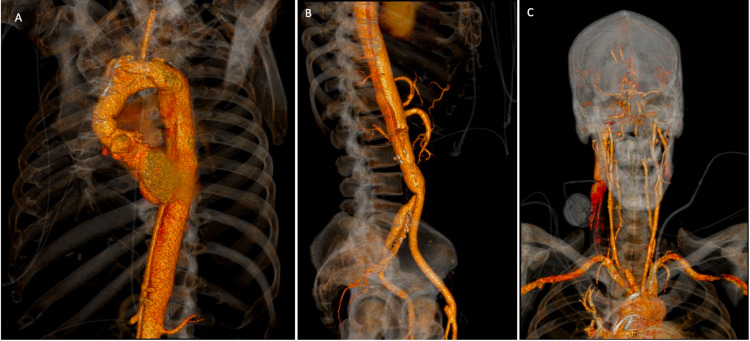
Three-dimensional computed tomography angiographic reconstruction showing extensive aortic dissection of the (A) ascending, descending, and (B) abdominal aorta. Panel C shows the extension of the dissection into the brachiocephalic artery and proximal aspects of the left common carotid artery and left subclavian artery.
